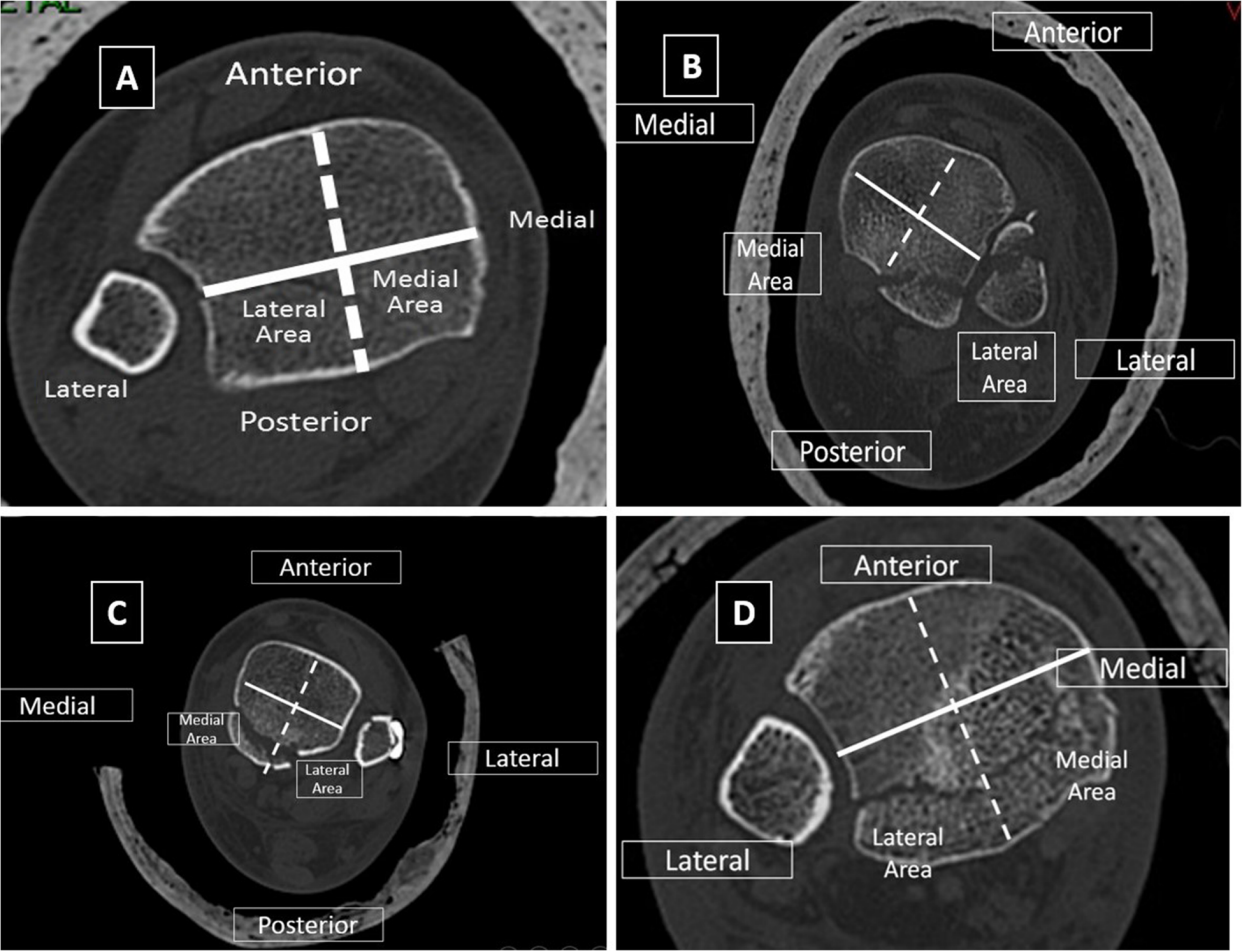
A method for CT-based classification of posterior malleolar fractures. a Superimposed on an axial CT image of the ankle, a solid line is subtended between the medial and lateral malleoli. Then, a second (dashed) line is drawn perpendicularly to the first intermalleolar line, dividing the posterior malleolus area into medial and lateral segments. b–d CT images showing the different fracture types: postero-lateral (b), postero-medial (c), and postero-central (d)
